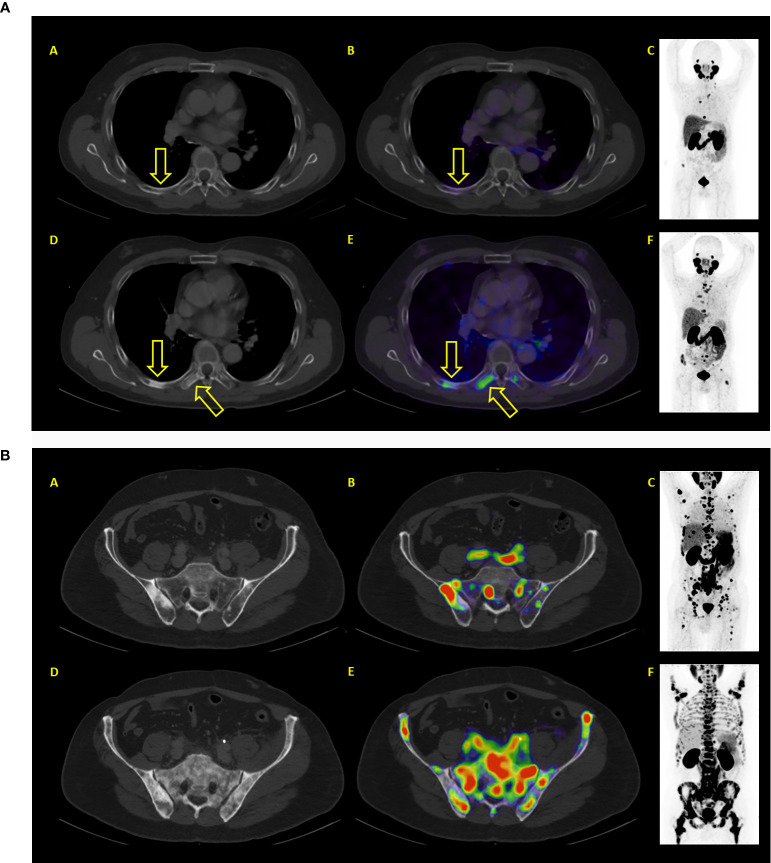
(A) Example of a patient where both PSA and PET showed similar results: progressive disease. After two therapy cycles PSA values increased from 93.2 to 214.2 ng/ml. PET/CT showed new bone lesions as well progression of previously known lesions. (A–C) represent baseline images whereas (C–E) represent interim PET/CT. (A, D): axial CT slices; (E): axial fused PET/CT images; (C, F) maximum intensity projection images. (B) Example of a patient where both PSA and PET showed similar results: progressive disease. After two therapy cycles PSA values increased from 327 to 1467 ng/ml. PET/CT showed diffuse new bone lesions. (A–C) represent baseline images whereas (C–E) represent interim PET/CT. (A, D): axial CT slices; (B, E): axial fused PET/CT images; (C, F) maximum intensity projection images.
